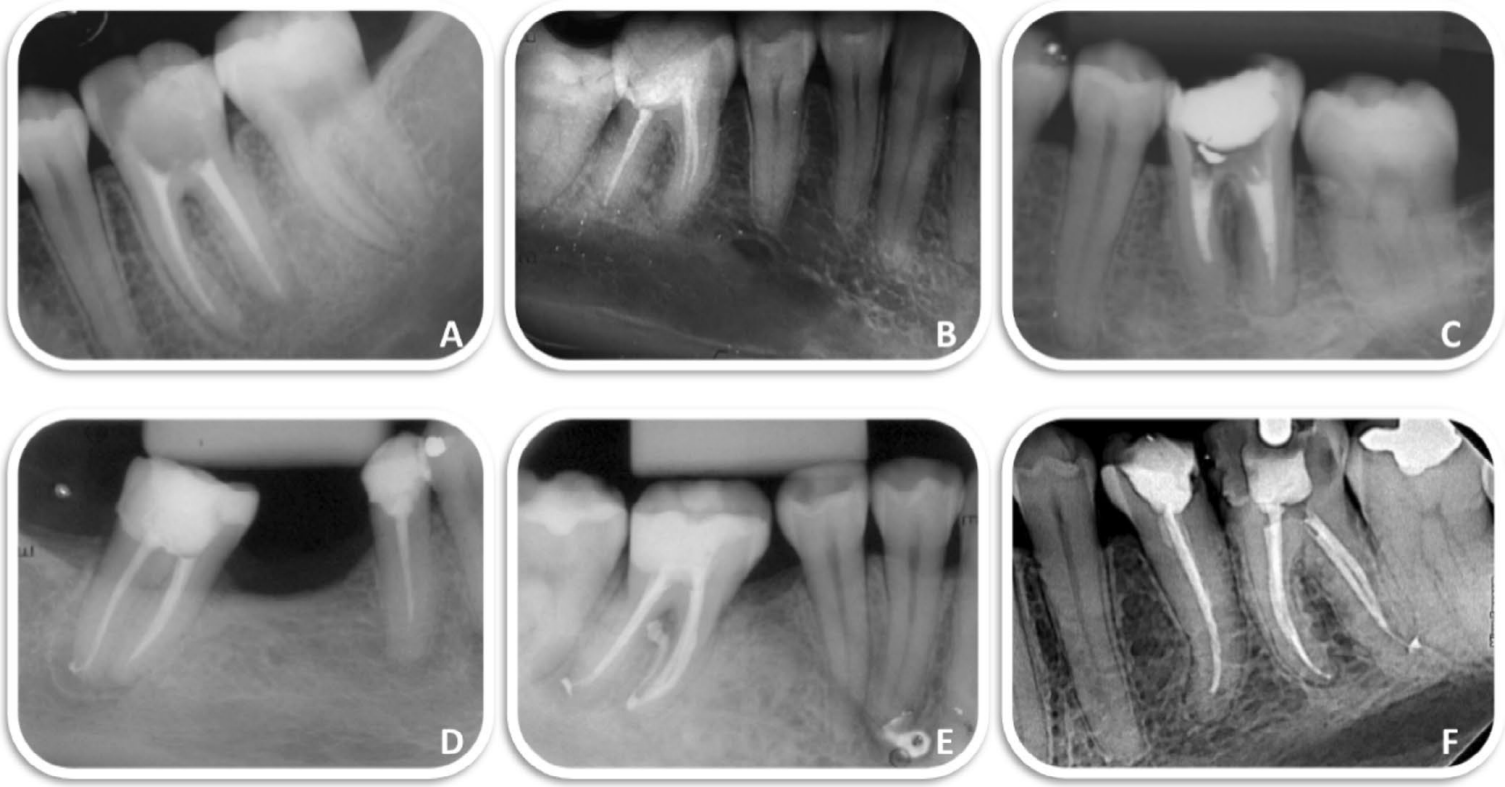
Post‐operative radiographs demonstrating treated canals using (A–C) manual technique and (D–F) reciprocating technique. The reciprocating technique exhibits superior maintenance of working length and root canal curvature, with greater respect for anatomical structure and reduced dentine loss. Obturation appears more homogeneous, though with occasional overfilling. In contrast, the manual technique resulted in loss of the working length and deviations from root canal curvature.
